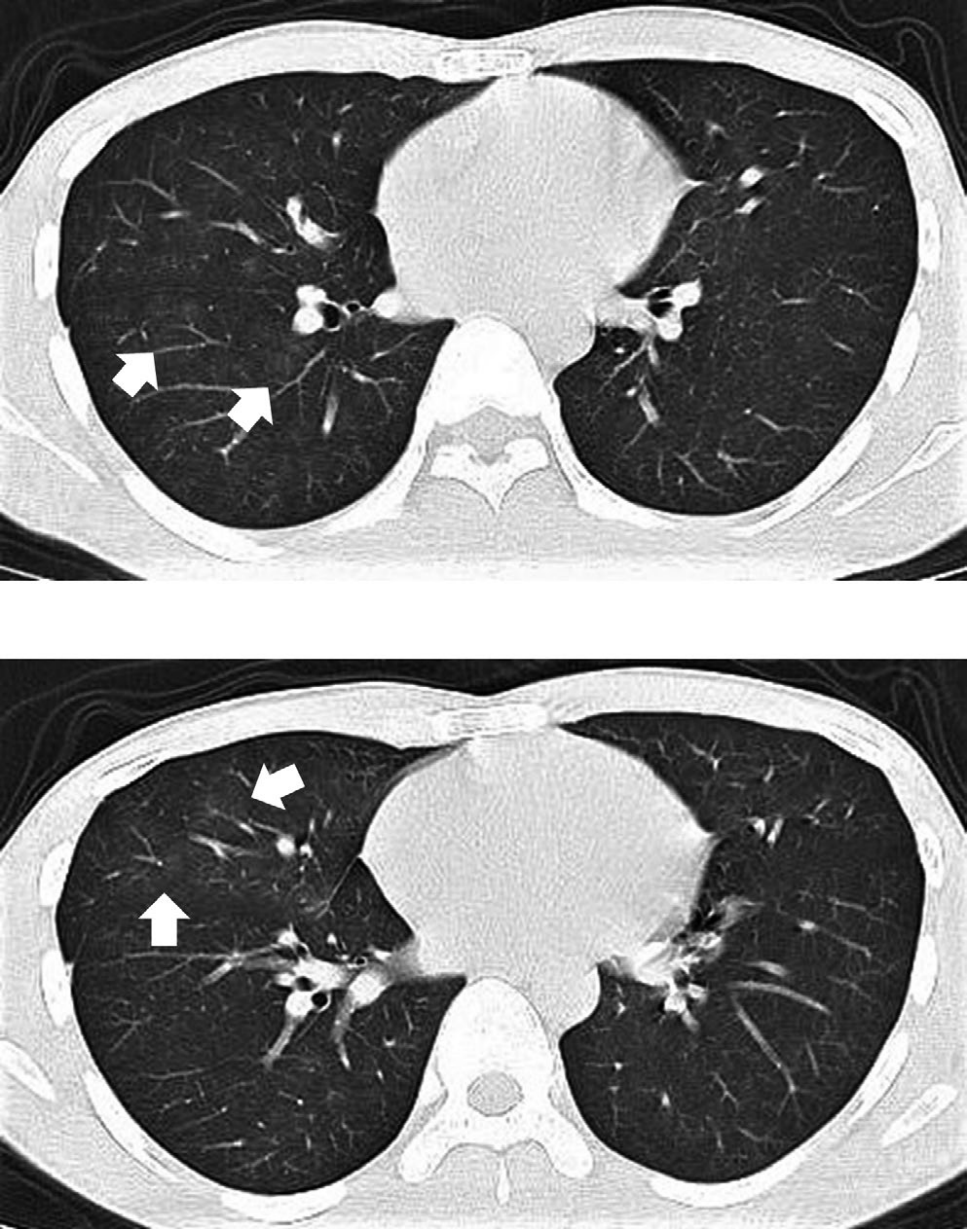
Chest CT at the initial visit. Patchy ground glass shadows (arrow) were observed in the right middle and lower lobe
